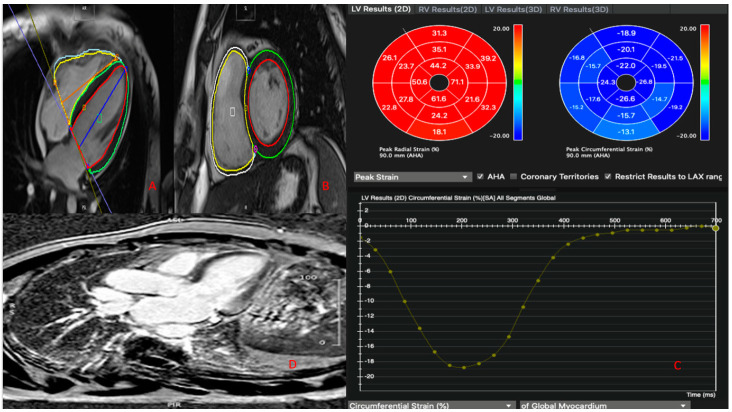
(A) Apical four-chamber CMR view. (B) Short-axis CMR view. The red and yellow lines trace the telediastolic endocardial border of the left and right ventricle, respectively. The green and white lines trace the telediastolic epicardial border of the left and right ventricle, respectively. (C) Two-dimensional LV radial and circumferential strain. The bullseyes show the average radial (red bullseye) or circumferential (blue bullseye) values for each of the 16 segments of the myocardium. The bottom of the figure shows the myocardial deformation curve. (D) Long-axis CMR view showing LGE.
